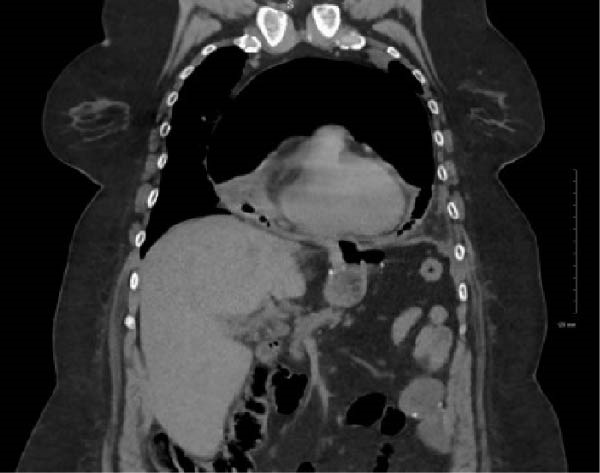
Coronal slice from CT imaging demonstrating large loculated hydropneumopericardium.
